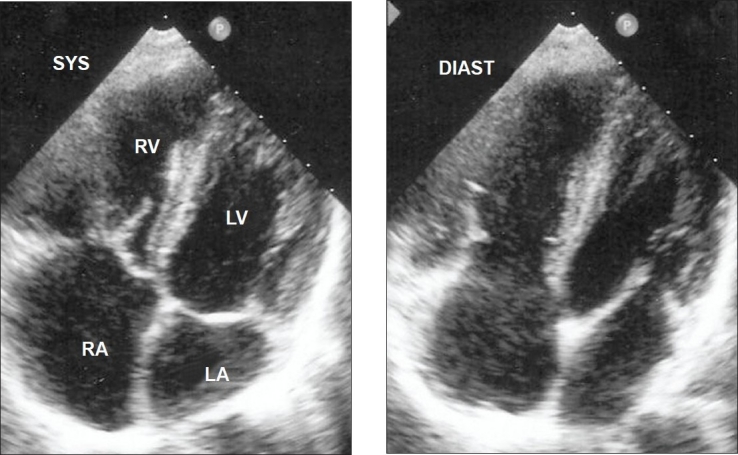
Transthoracic echocardiogram showing complete resolution of right atrial thrombus with oral anticoagulation in a patient with polycythemia rubra vera, splanchnic vein thrombosis and mobile right heart thrombus. Abbreviations as before.
